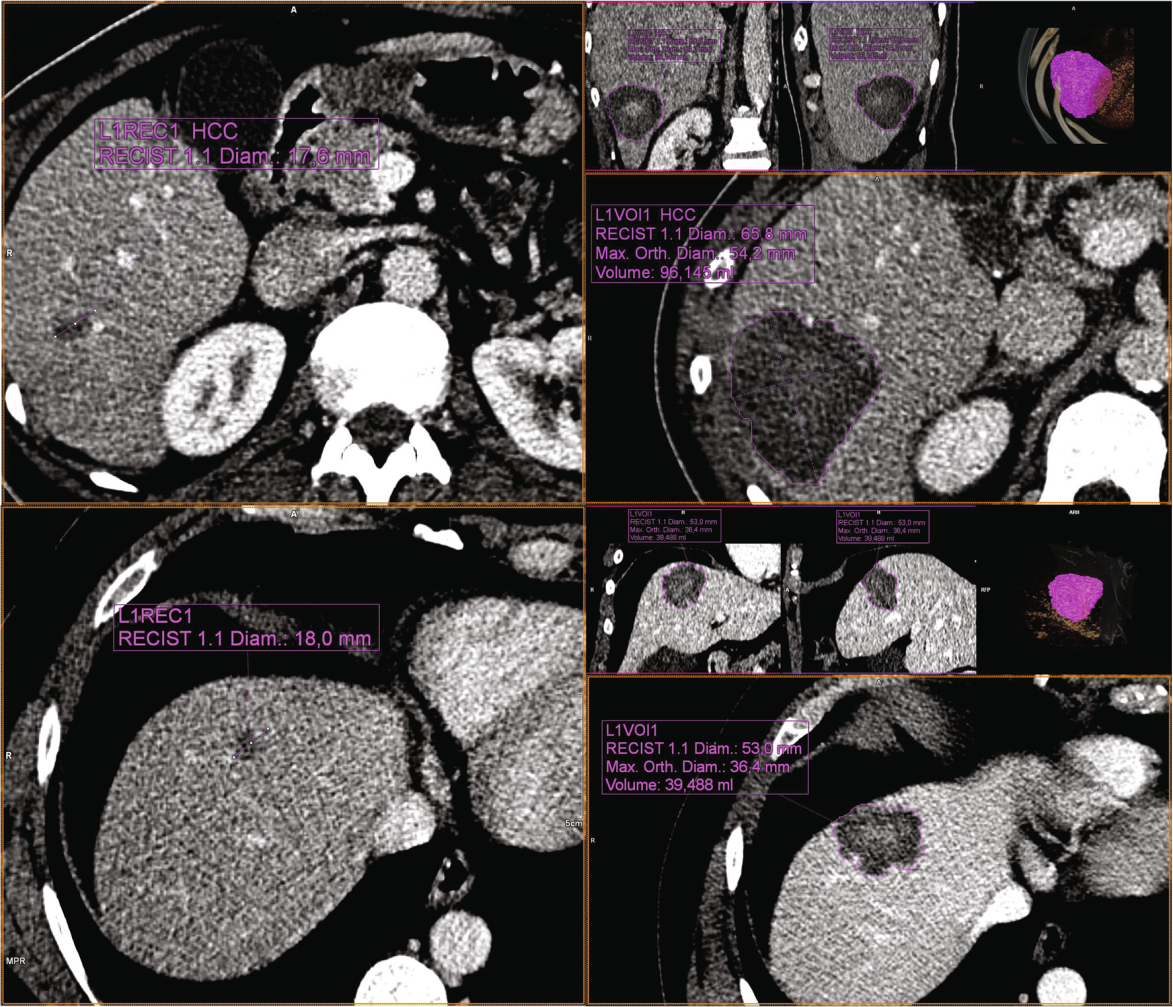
Left* Preprocedural portal venous phase contrast enhanced CT images of HCC in segment 6 (*top*) and CRLM in segment 4 (*bottom*) in two patients who had not received systemic therapy or transarterial (chemo)embolization. Using MWA device B, 96 kJ (100 W for 8:00 min × 2) and 96 kJ (100 W for 6:00 min, 100 W for 10:00 min) were applied to the HCC and CRLM, respectively, with 16 mm and 14 mm between the two positions of the ablation center of the antenna, so overlap was approximately similar. *Right* Resulting ablation zones after segmentation on the 1-week follow-up portal venous phase contrast-enhanced CT images using the MM Oncology package (*syngo*.via; Siemens, Erlangen, Germany), with ablation zone volumes of 96 mL and 39 mL, resulting in energy deposition ratios of 1.00 mL/kJ and 0.41 mL/kJ for HCC and CRLM, respectively. After 6 months of follow-up, the HCC showed no sign of recurrence, whereas a PET scan of the CRLM showed activity at the dorsal side of the ablation zone, for which re-ablation was performed
